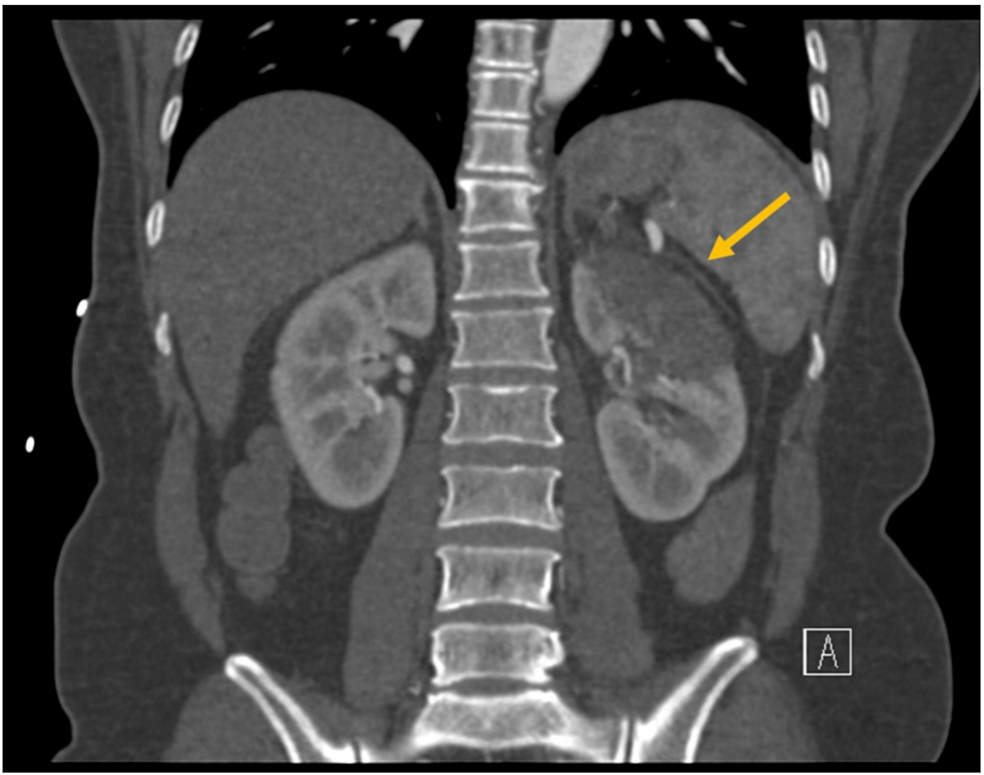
CT abdomen revealing left upper pole cortical hypoattenuation compatible with infarct with left renal segmental artery occlusion.
